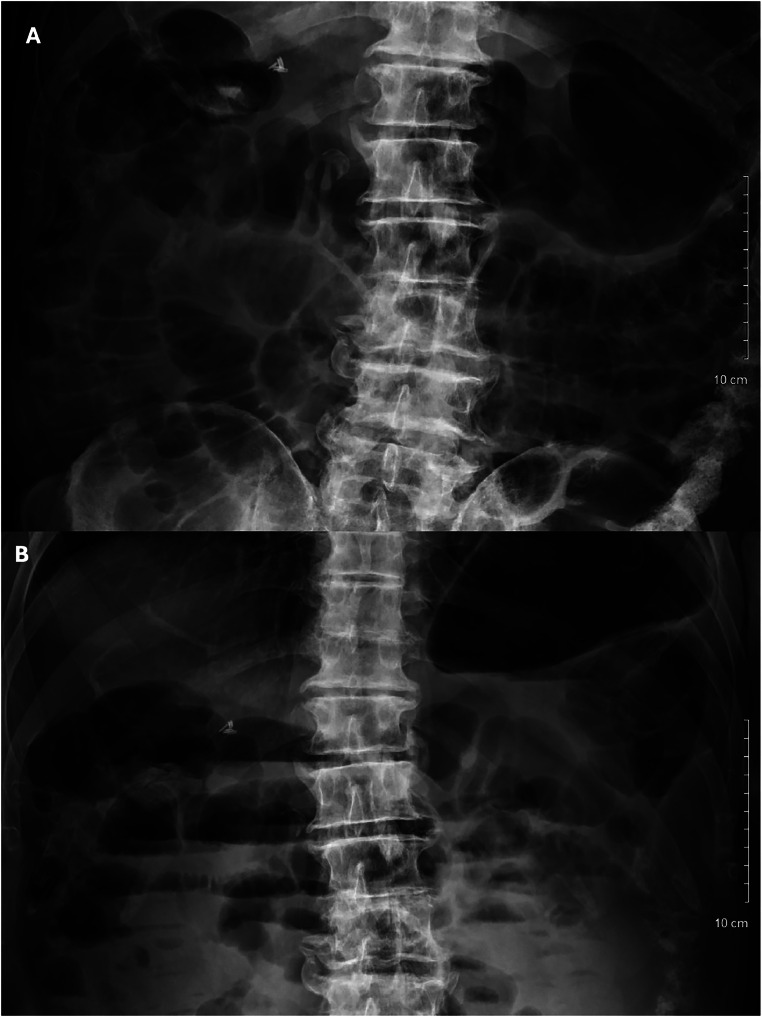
Supine (A) and upright (B) anteroposterior abdominal radiograph upon readmission showing gaseous distention of multiple loops of small bowel with air-fluid levels.
